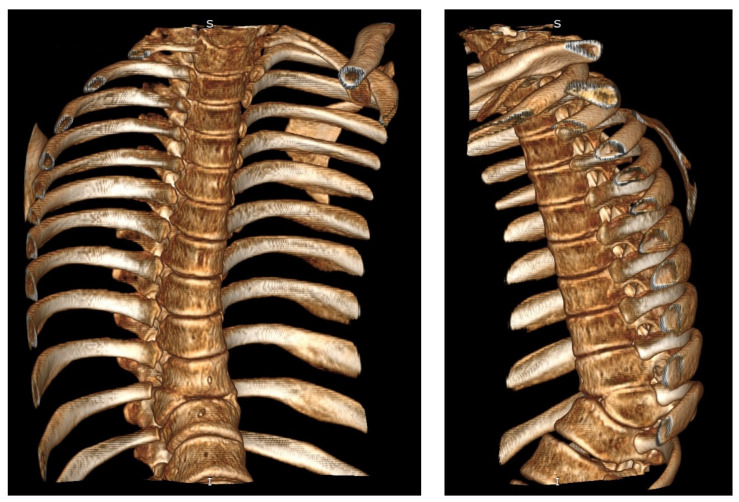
3D computed tomography reconstructions shows the butterfly vertebra of Th10 driving the kyphoscoliotic deformity in a 15-year-old boy.
